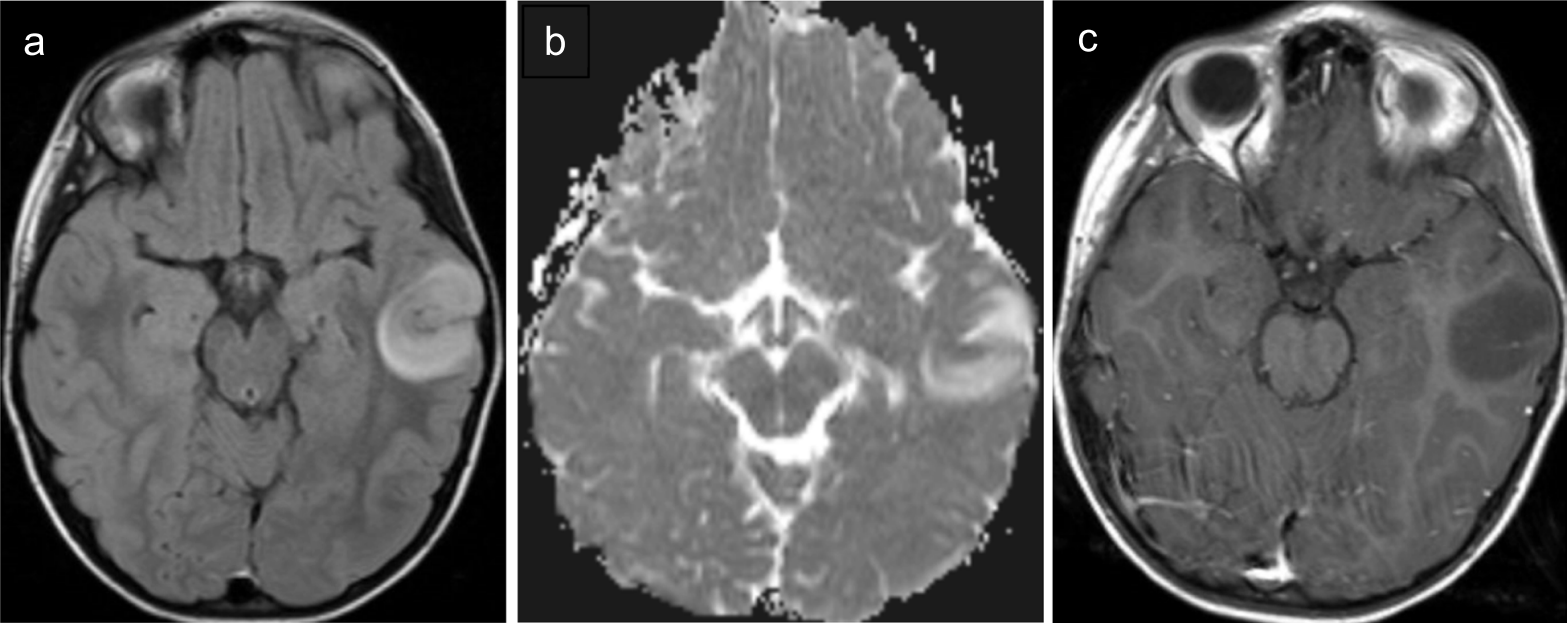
Diffuse astrocytoma *MYB/MYBL1*-altered in a 7-year-old female identified incidentally after a go-cart accident. Axial T2 FLAIR (a), ADC (b), and T1-weighted contrast-enhanced (c) MR images show a circumscribed, cortically-based, mildly expansile, heterogeneous, FLAIR-hyperintense left temporal mass with facilitated diffusivity and no internal enhancement
